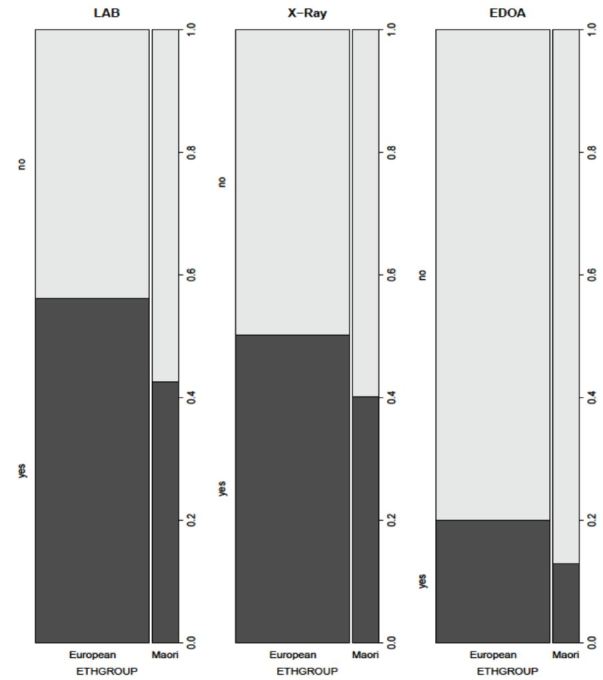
Proportion of Maori and European patients admitted to our emergency department observation area (EDOA), had blood tests performed (Lab), or had radiographs performed.
